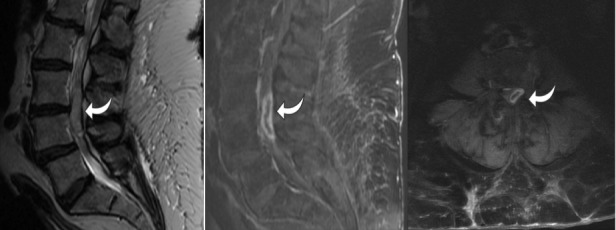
Preoperative Lumbar Spine MRI T2 sagittal, T1 sagittal contrast-enhanced, and T1 axial contrast-enhanced MRI of the lumbar spine showing a 30 mm × 5 mm × 7 mm L3-4 extradural mass that is mildly T2 hyperintense and T1 hypointense with a thick rim of contrast enhancement (arrow)
